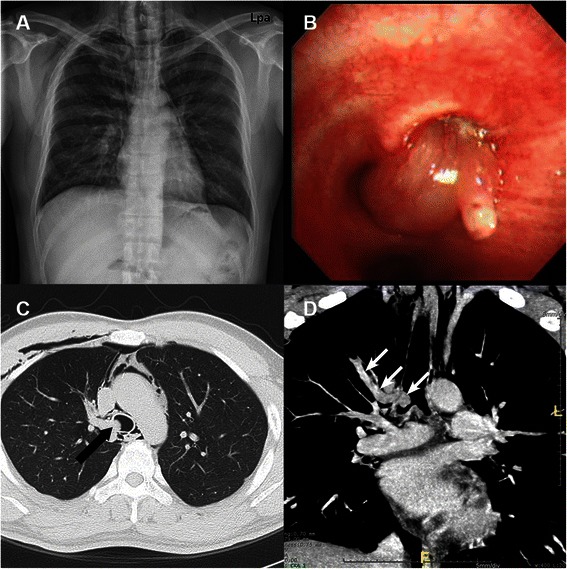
a Initial chest X ray shows pneumomediastinum. b Bronchoscopy finding of a protruding and glistering tumour originating from the right side of the trachea. c Computed tomography showing an elongated endobronchial tumour in the accessory tracheal bronchus originating from the right side of the lower tracheal wall (black arrow, axial view). d Computed tomography showing a tumour located in the right upper lobe from the accessory tracheal bronchus (white arrow, coronal view)
